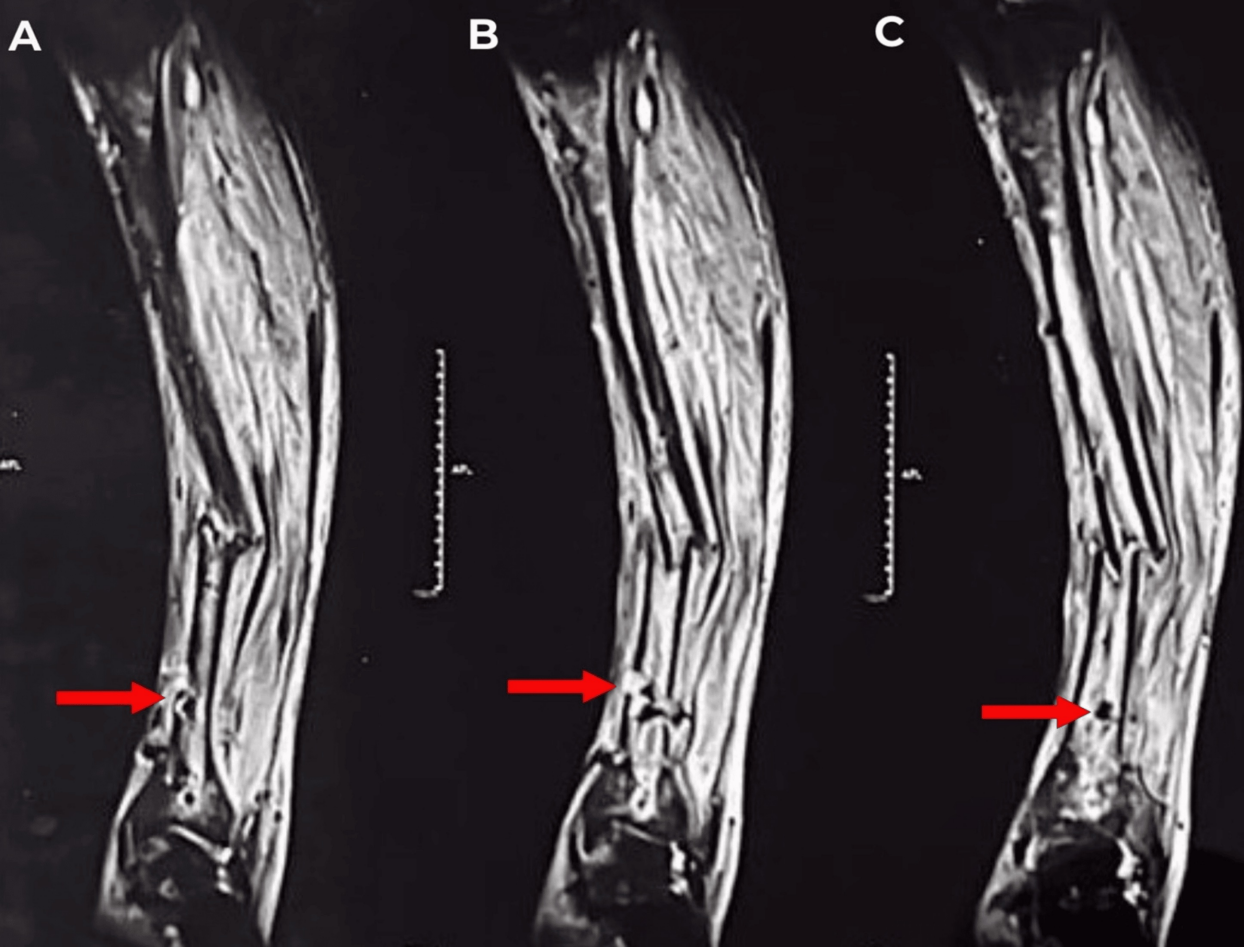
A-C) The sagittal section of the left leg MRI. Red arrows show a cortical breach in the lower one-third part of the tibia, through which a collection of fluid was seen. MRI: magnetic resonance imaging
